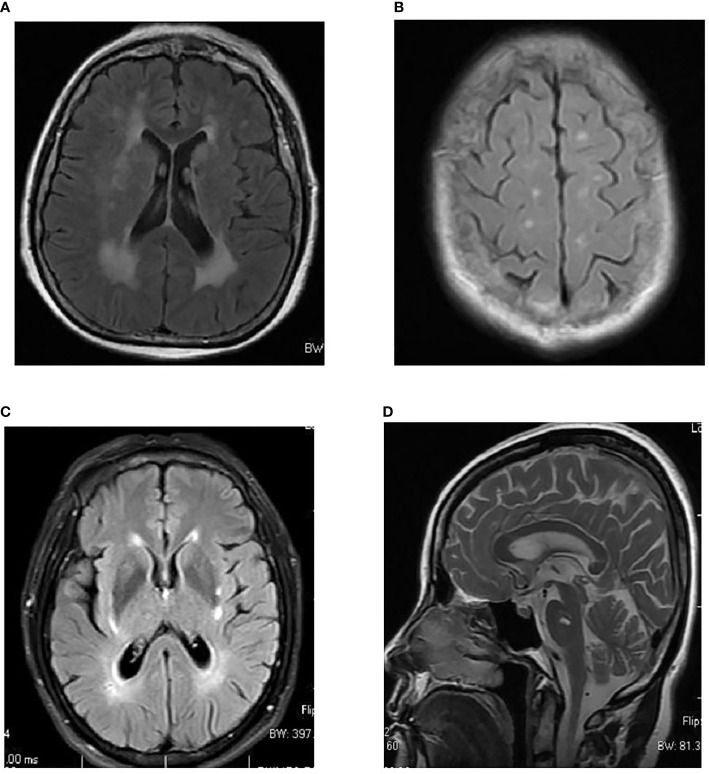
Examples of topographical distribution of white matter lesions in MRI. (A) Periventricular white matter lesions (WMLs) (pvWMLs). (B) Subcortical WMLs (sWMLs). (C) Basal ganglia WMLs (bgWMLs). (D) Infratentorial WMLs (iWMLs).
